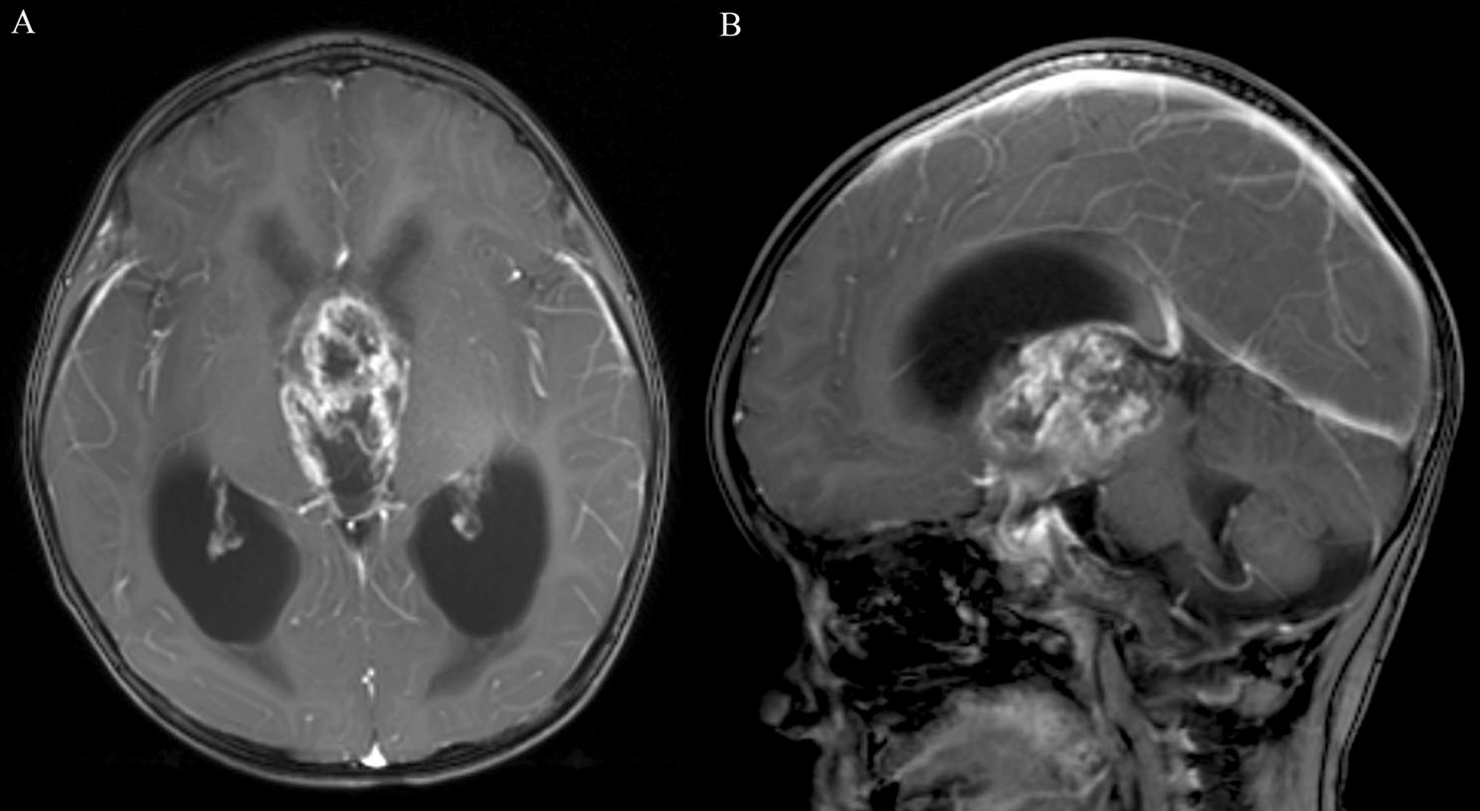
A 4-year-old patient with a suprasellar PA. Axial (A) and sagittal (B) postcontrast T1-weighted images show a peripherally ground-glass-like appearance enhancing lesion with crests on the inner edge. The lesion resembles the flesh of a longitudinal section of a cut green pepper with multiple indentations on the surface, and discrete point-like enhancement inside the tumor
